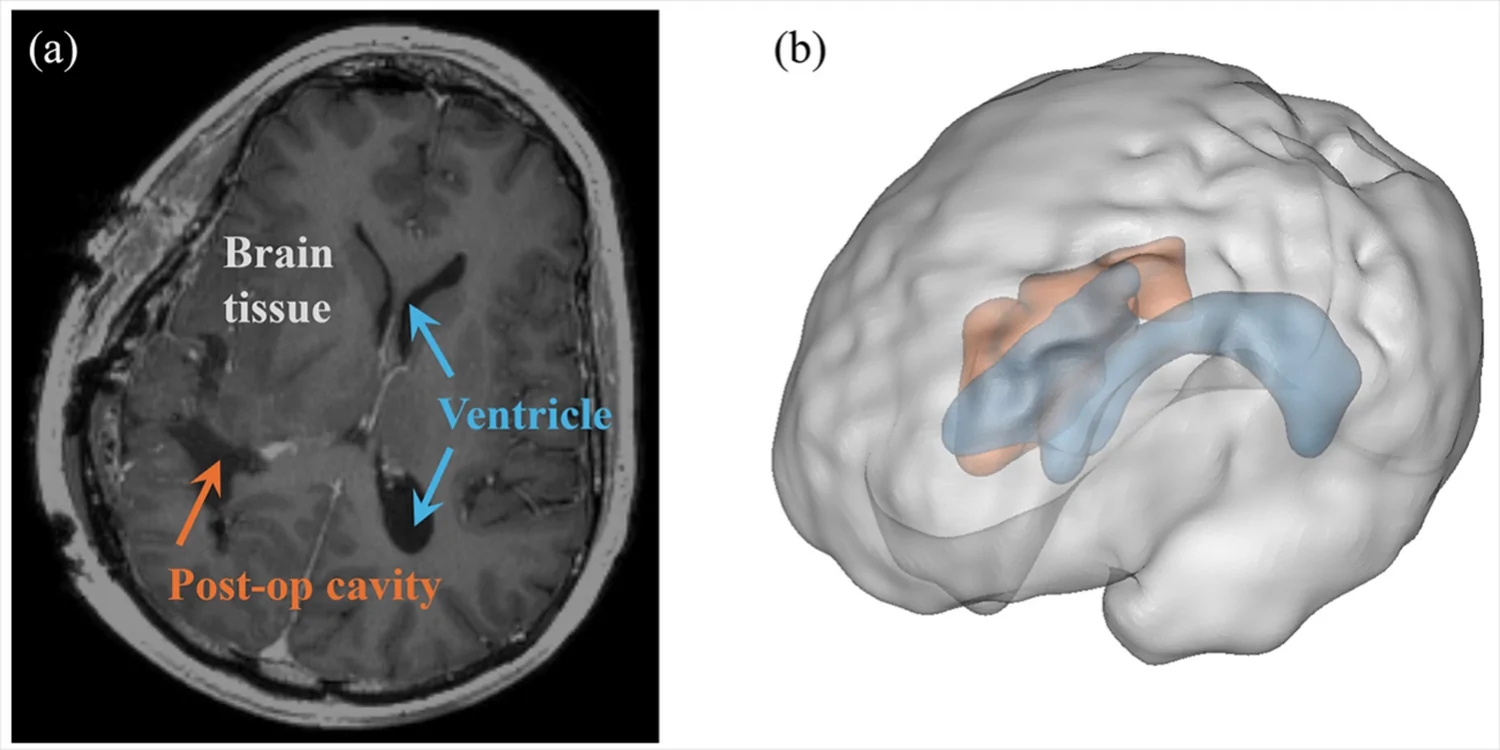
Model geometry. (a) An example MRI slice shows the brain parenchyma, ventricle, and post-surgery cavity. (b) the 3-D computational domain. The pale blue region is the brain ventricle. The orange region is the cavity left after the tumour resection and filled with the hydrogel, which is loaded with a nanocarrier-encapsulated drug.
